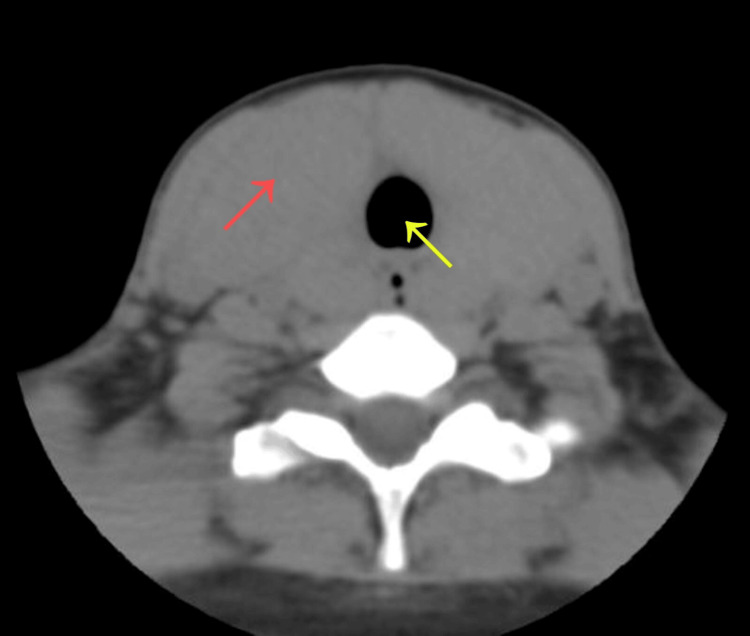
Axial CT scan of the neck showing diffuse enlargement of the thyroid gland (red arrow) causing tracheal compression and anterior displacement (yellow arrow).
